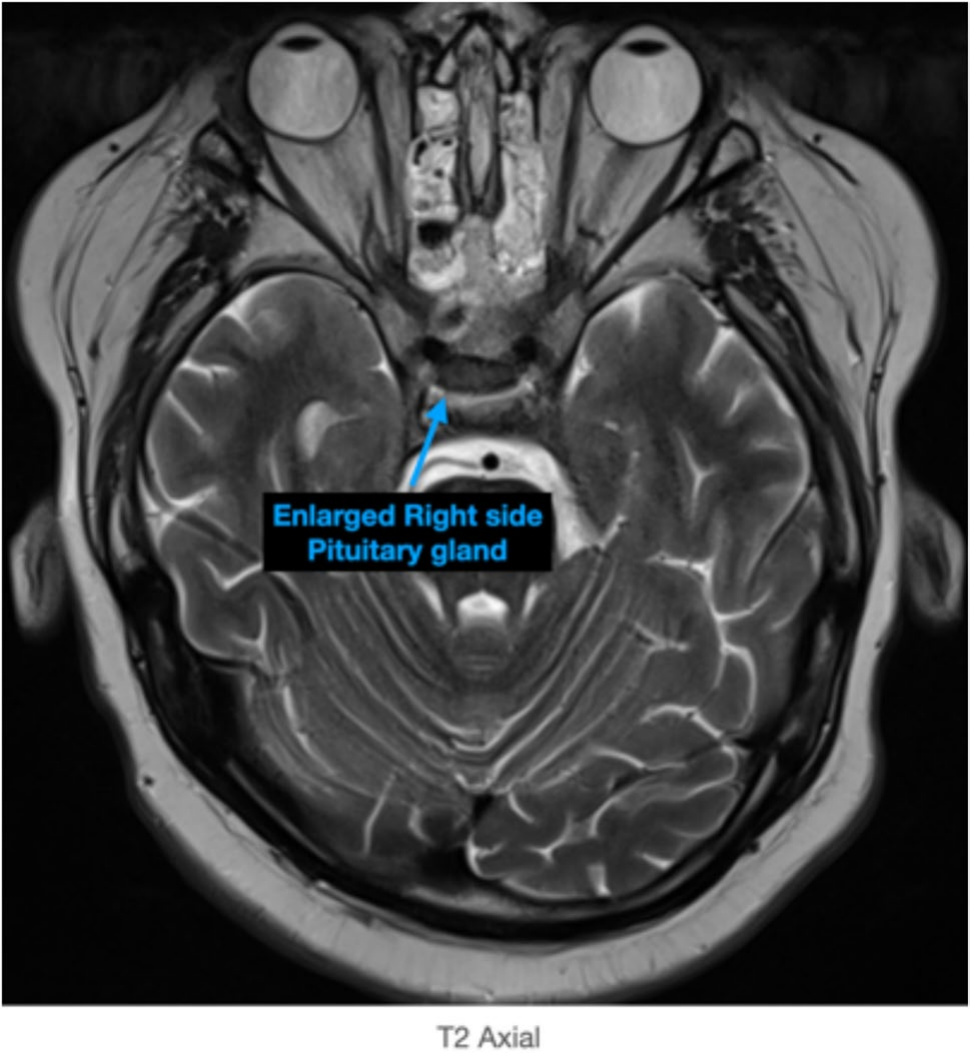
Axial MRI T2 demonstrates a right-sided pituitary gland enlargement correlating to the pituitary microadenoma
